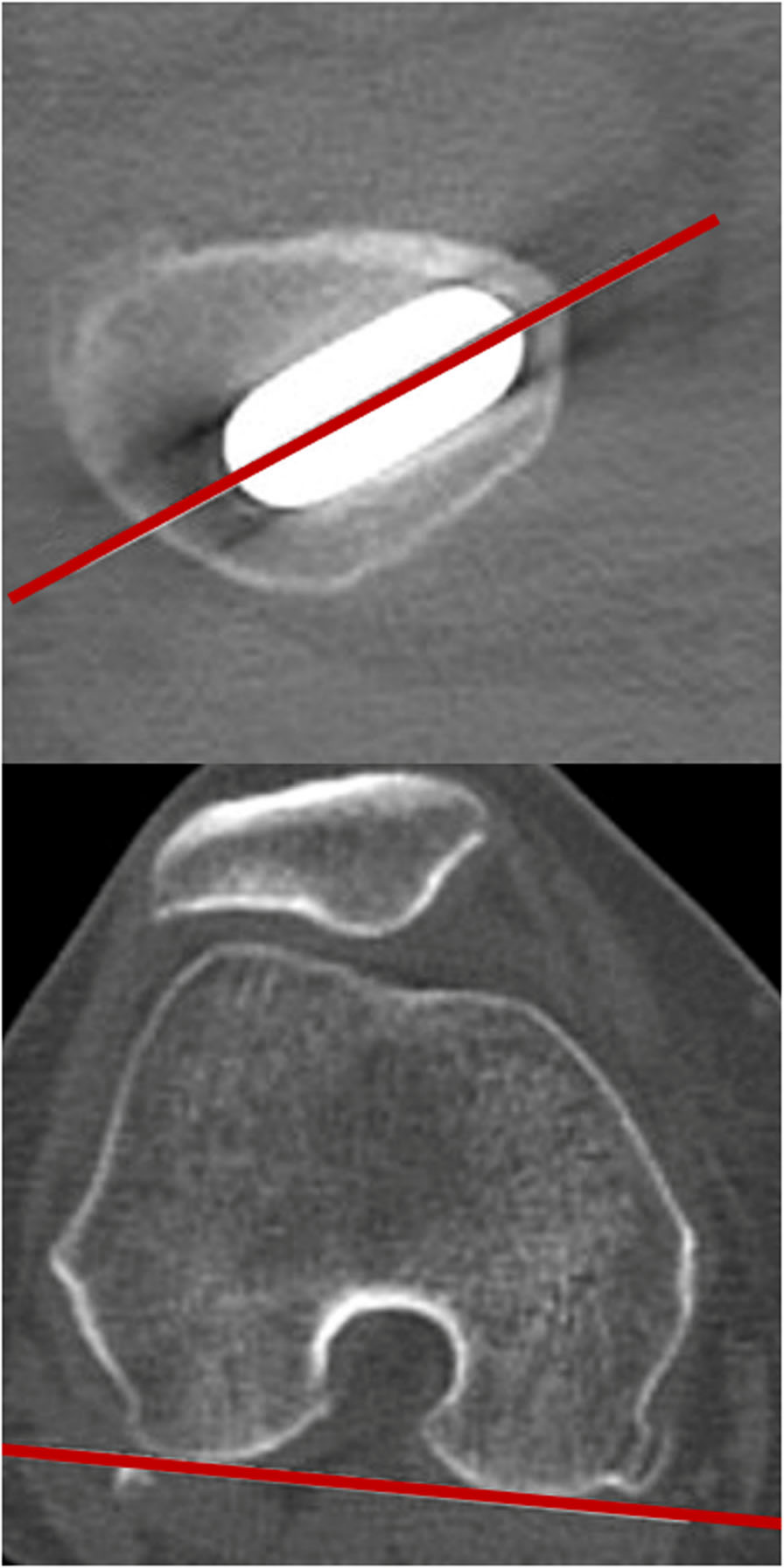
Measurement of stem anteversion on CT scan. The anteversion angle was measured as the angle between the stem-neck axis and an axis parallel to the posterior aspect of the femoral condyles. After measurement, the anteversion angle of the stem was 35°
